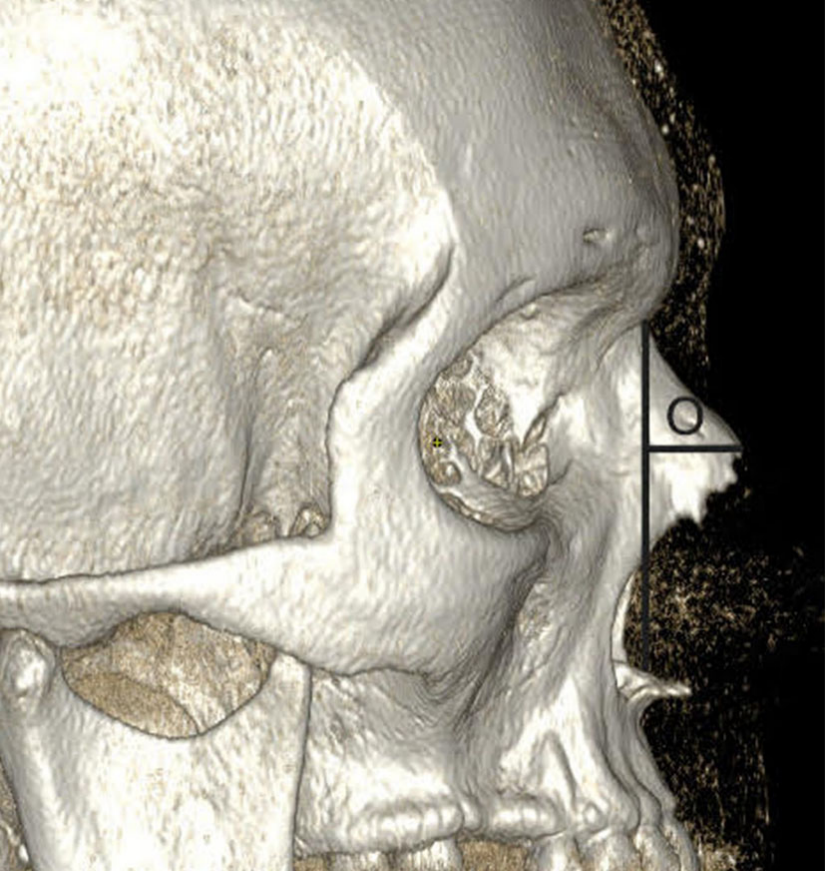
Three-dimensional CT reconstruction from the lateral view showing measurement of nasal bone height (O) in the V-shaped nasal bony vaults
